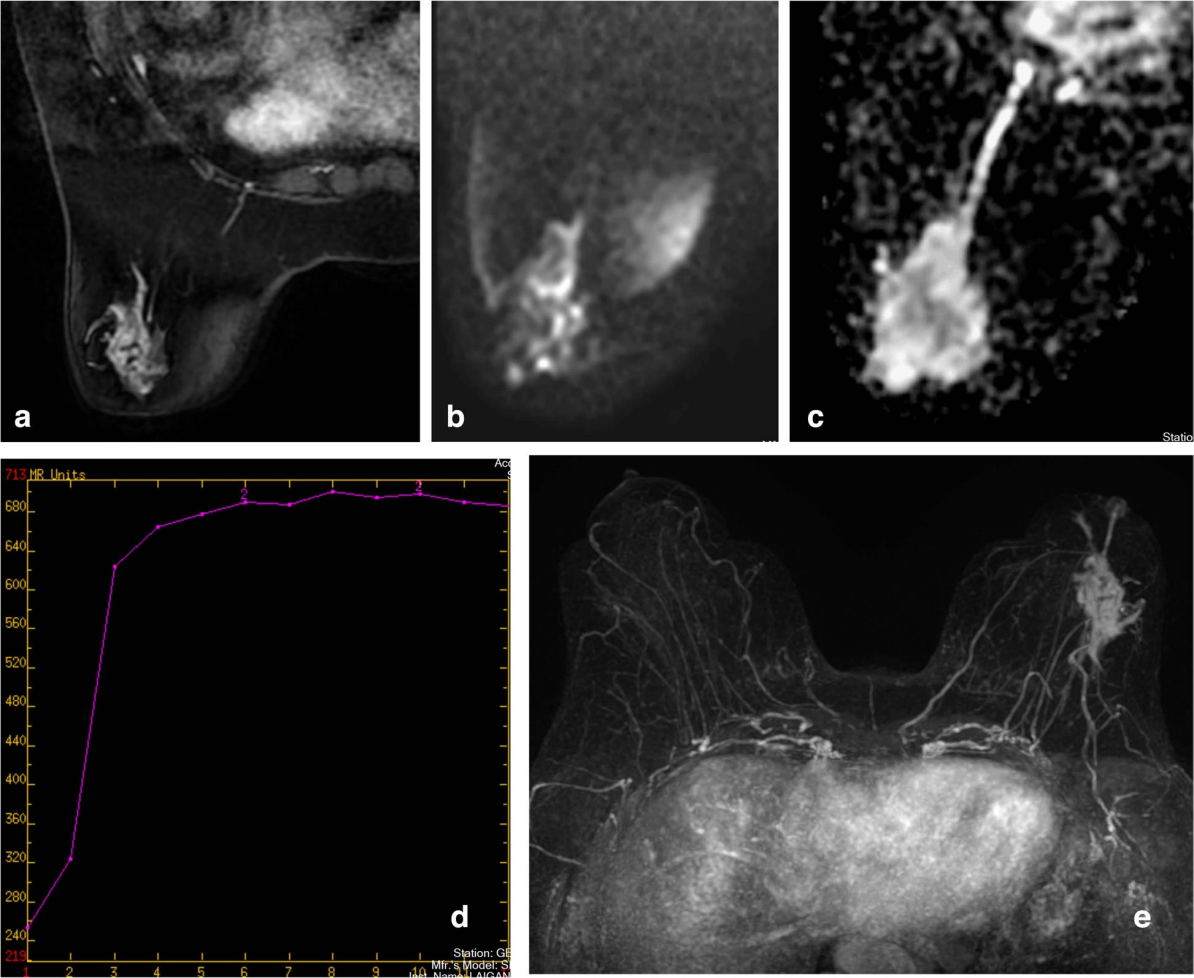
a A lesion in a 58-year-old female with segmental distribution and heterogeneous enhancement. b and c The ADC value was 1.58 × 10^–3^ mm^2^/s, d The imaging revealed an typeII TIC curve. e The imaging revealed feeding vessels. The multiparametric score was 4the lesion was determined as BI-RADS-MRI type 4c. The pathological finding was hyperplasia of the left breast with intraductal carcinoma in situ
